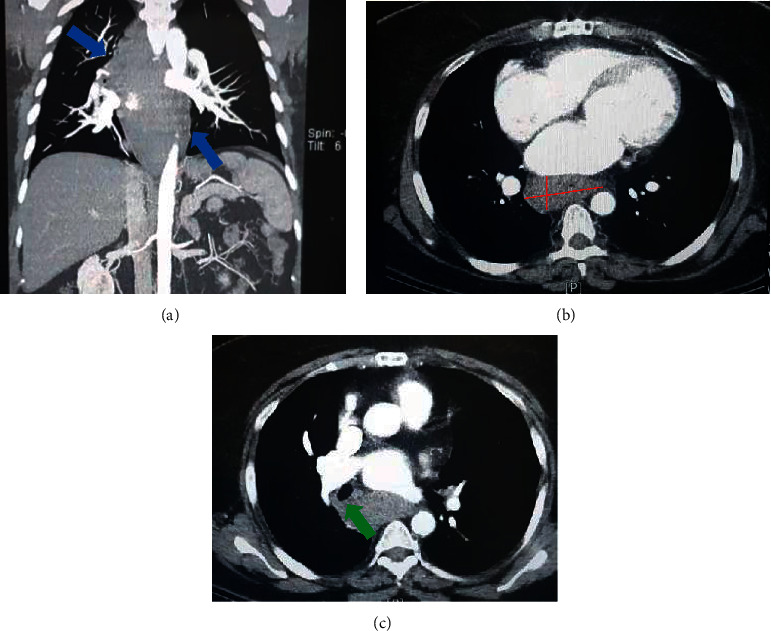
Contrast-enhanced axial and coronal computed tomography of the chest showing an abnormal esophageal contour beginning about 16 cm from incisura extending about 20 cm, terminating on LES ((a), blue arrows). The CT images clearly show esophageal dilatation with a maximum lesion size of 5 × 2.2 cm ((b), red lines), irregularity, and tracheal deviation caused by hematoma ((c), green arrow) *(date performed: December 21, 2021)*.
